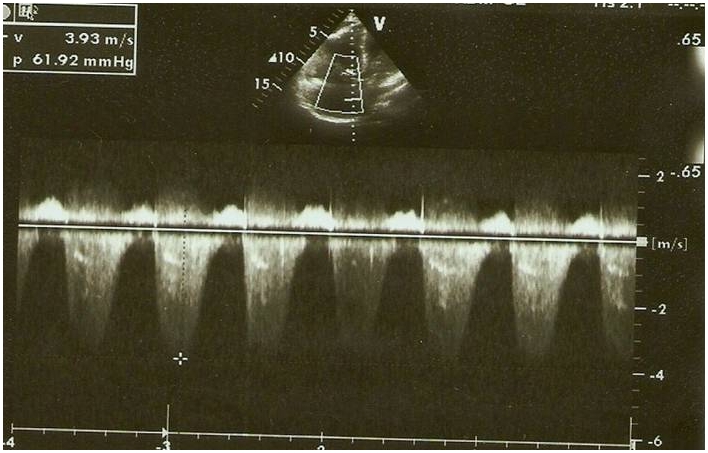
Image d’échocardiographie mode 2D, incidence 4 cavités associée au Doppler continu mettant en évidence une HTAP sévère (PAPs à 66 mmHg)
